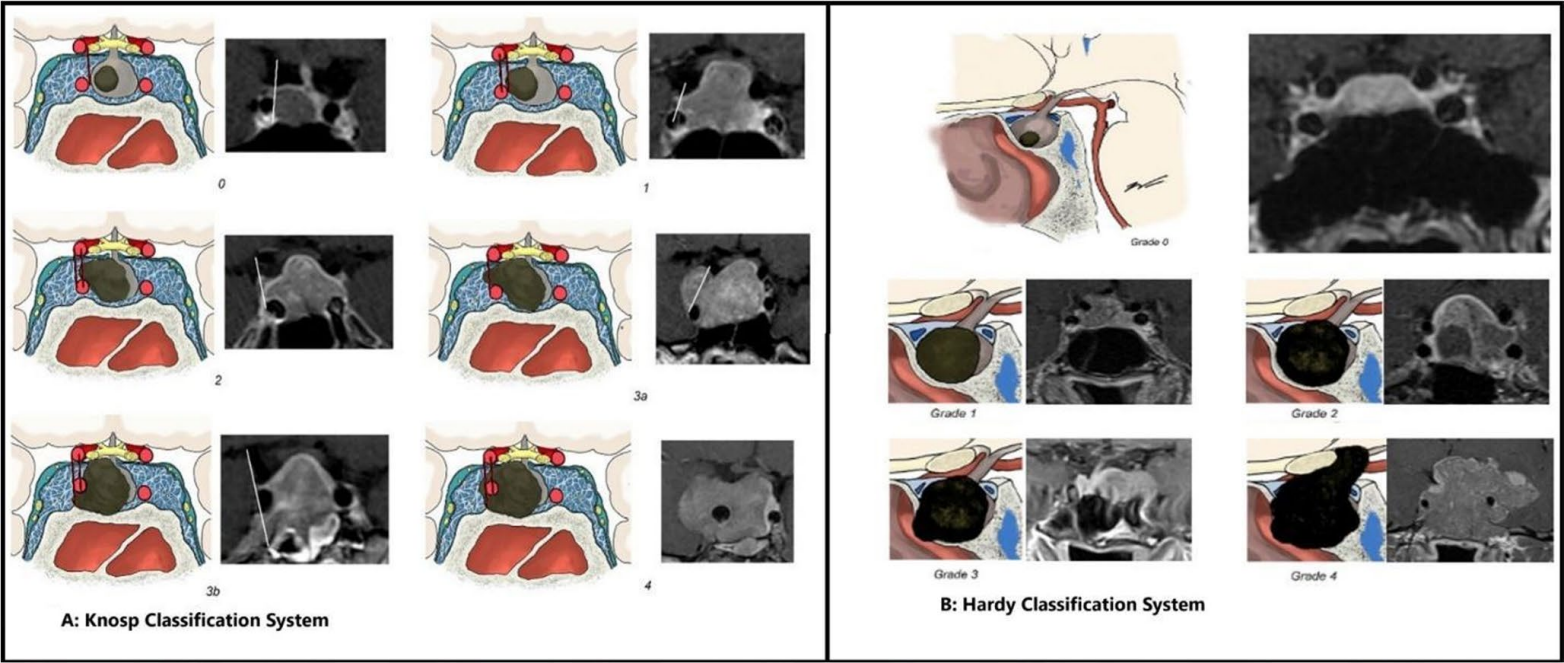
A visualization of the stages of the Knosp and Hardy classifications and accompanying MRI images
